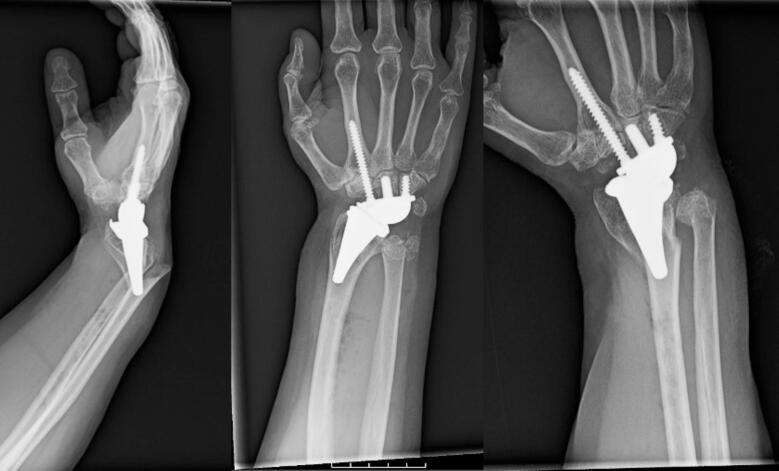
Right wrist radiographs on presentation to the Emergency Department prior to closed reduction demonstrating peri-implant distal 1/3rd radius fracture and distal ulna fracture. Of note, the radial component appears well fixed in the distal bone.
